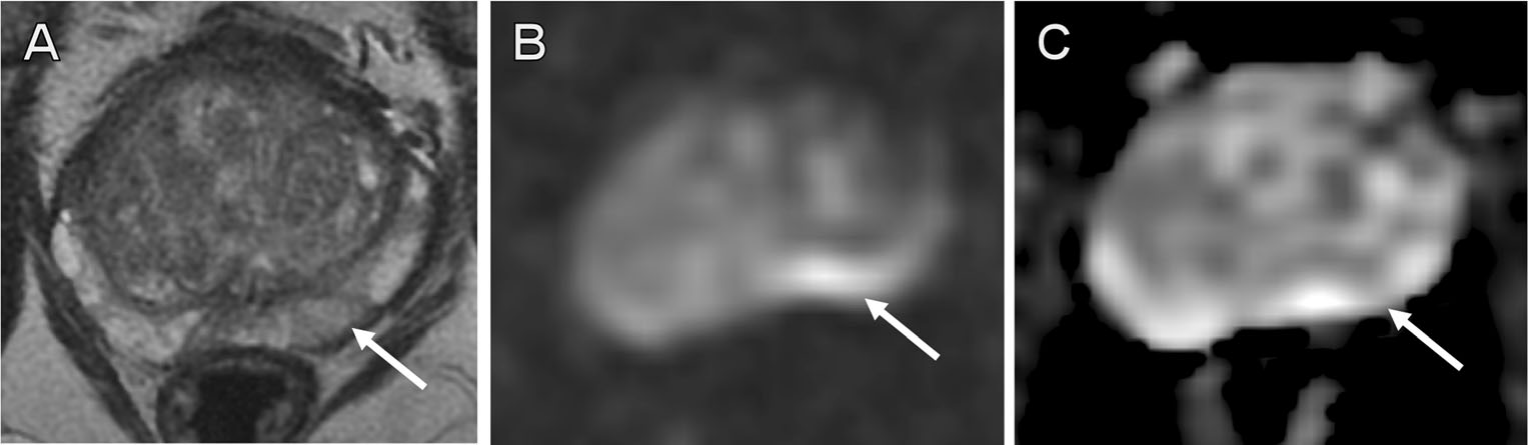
False-positive external report. A 64-year-old patient with 7.1 ng/ml PSA and previous negative TRUS biopsy. External report described a high probability target in the left mid peripheral zone. Second read called a negative MRI, with linear areas of intermediate T2 signal in the left mid (a, PIRADS-2) and high signal on *b*-1,400 imaging (b) thought to be artefactual due to rectal gas, and without convincing low signal on ADC maps (c). Subsequent transperineal template biopsy showed all 24 cores to be benign
